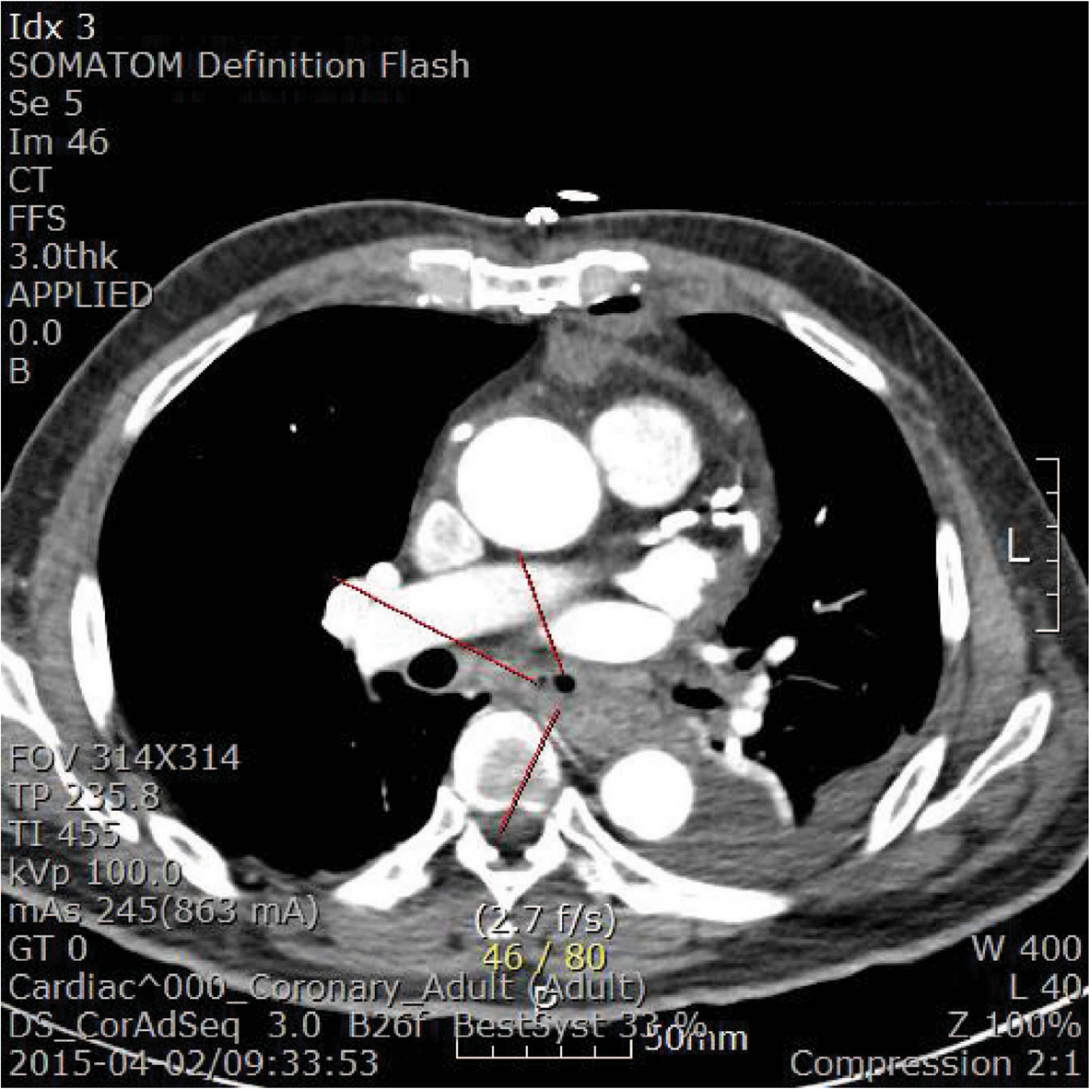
Chest computed tomographic scan. Abscess formation or air-containing tissue in retrosternal, pericardial, and paraesophageal areas is seen. Red lines indicate esophageal wall thickening
